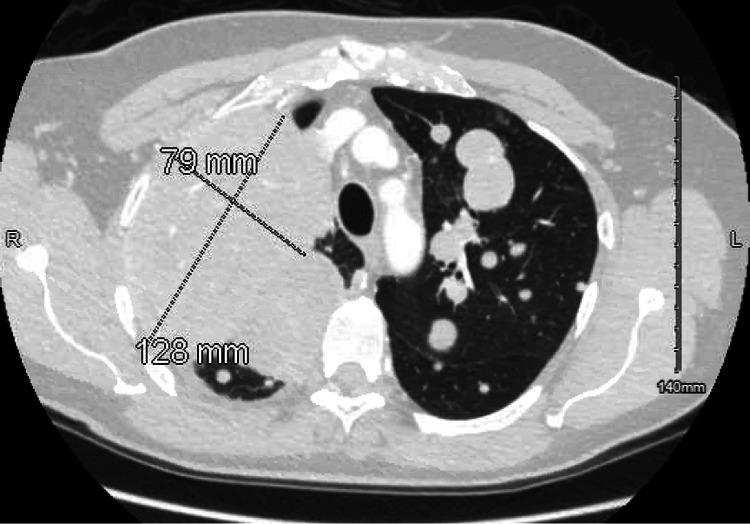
CT scan of the chest showing an 8 × 4.5 cm subcarinal mass concerning for metastasis of the solitary fibrous tumor
